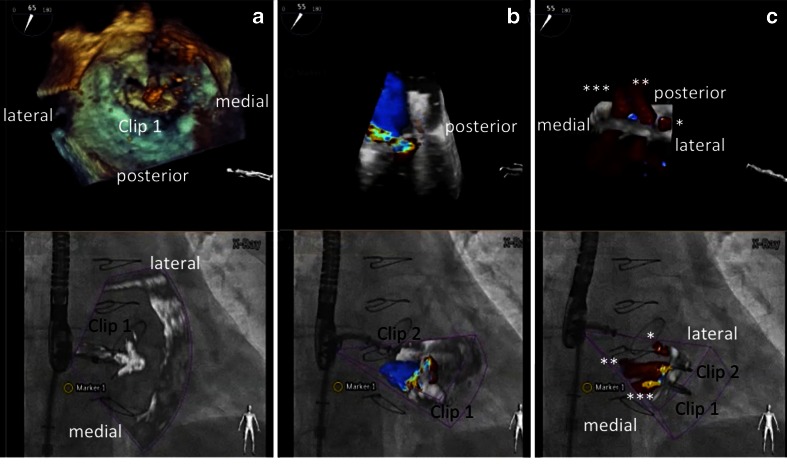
Overlay of 3D real-time echocardiography and fluoroscopy during a MitraClip procedure. a–c demonstrate step by step the insertion of clip 1 and 2 (each with 3D real-time echo on *top*, fusion image at the *bottom*). a Orientation of the clip relative to the mitral valve leaflet margin after clip opening. b Residual moderate mitral regurgitation after insertion of clip 1 in the medial part of the segments two. A second clip is approaching the valve. c Residual mild mitral regurgitation with three jets (denoted with *single*, *double*, and *triple asterisks*) after the insertion of the second clip
